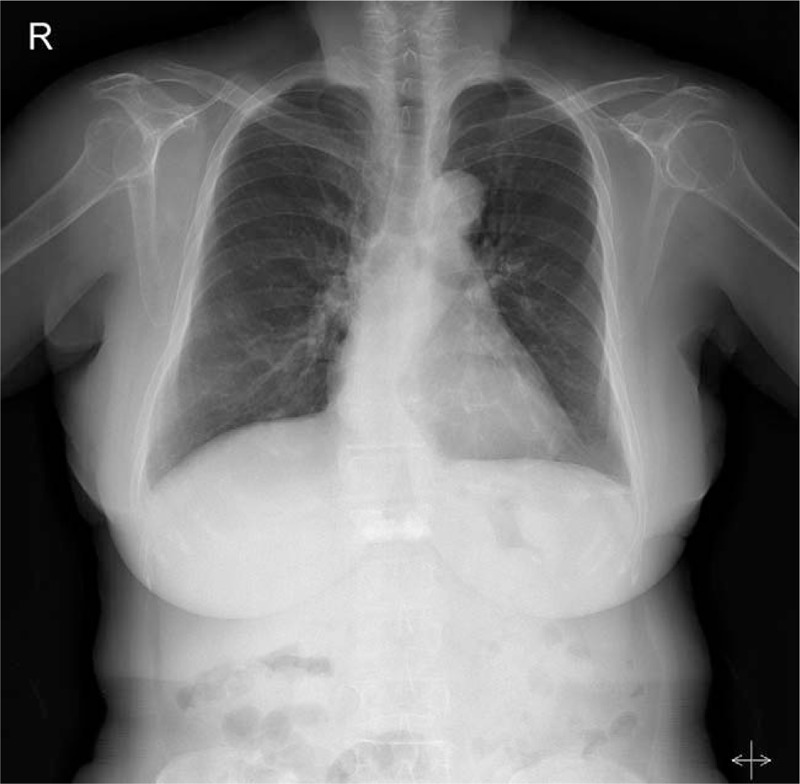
Preoperative chest X-ray shows no specific lesion.
